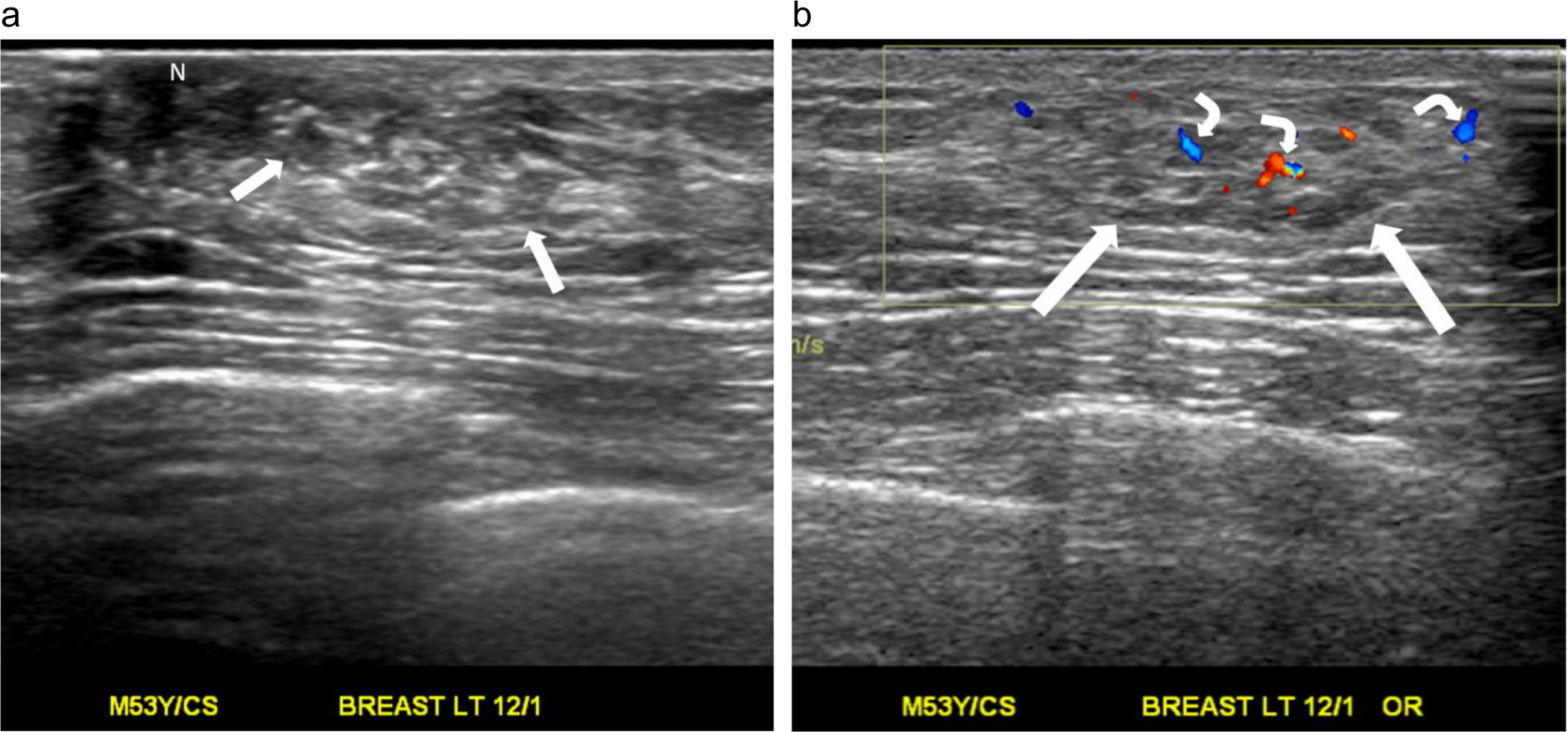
A palpable lump in the left breast of a 53-year-old man. (a) Radial ultrasound shows an irregular shape, a noncircumscribed margin, and a heterogeneously isoechoic, solid nodule (arrows). The nodule has a parallel orientation and a markedly eccentric relationship to the nipple (N). (b) Color Doppler ultrasound shows occasional transient color pixels and multiple short pedicles of blood supply (curve arrows) in the nodule (straight arrows), classified as a rich color flow signal with predominantly internal vascularity. The postoperative pathological diagnosis is gynecomastia.
